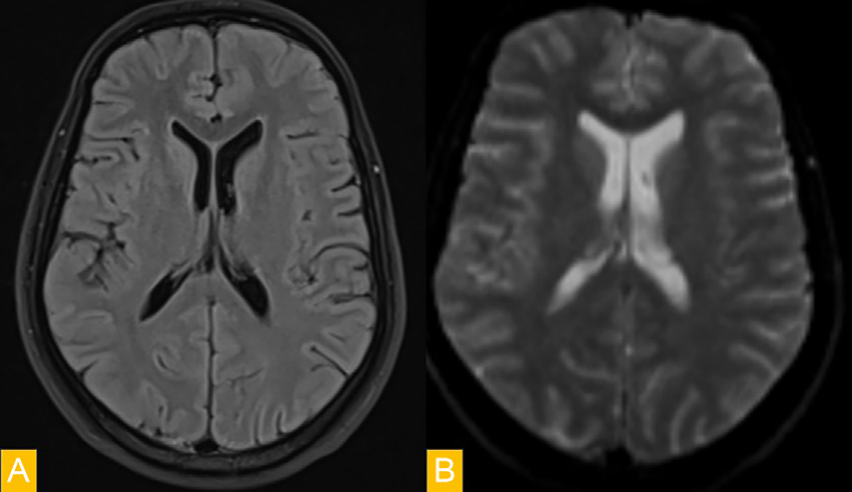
Follow-up MRI demonstrating complete resolution of the splenial lesion. No abnormal signal is seen on FLAIR (A) or diffusion-weighted imaging (B), confirming the transient nature of the lesion.
